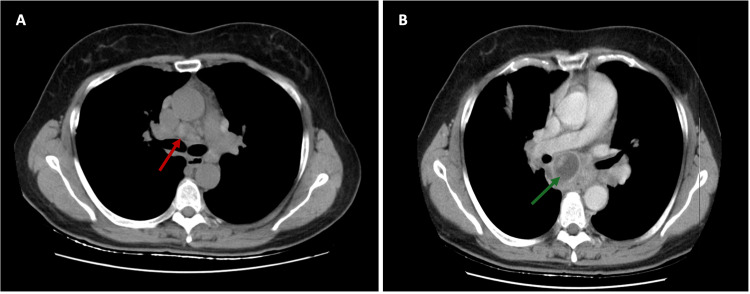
Chest CT images (axial view) showing (A) Subcarinal adenomegaly with 17 mm in its short axis before EBUS-TBNA (red arrow) and (B) Posterior mediastinal abscess after EBUS-TBNA (green arrow). EBUS-TBNA: endobronchial ultrasound-guided transbronchial needle aspiration
